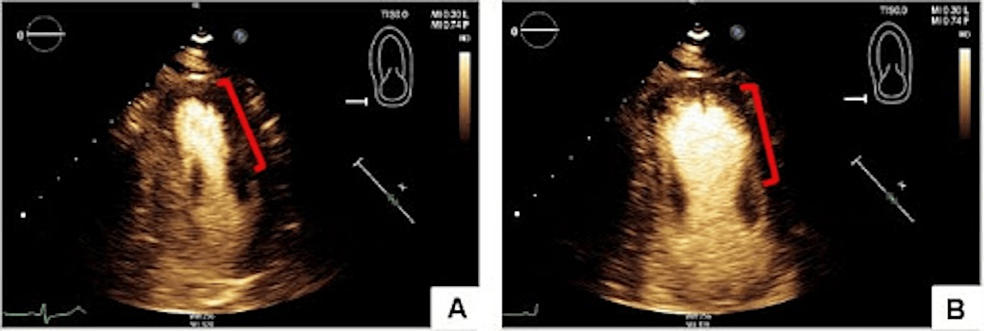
Echocardiography in apical view with perflutren lipid microsphere contrast demonstrating LV chamber in systole (A) and diastole (B). Note the LV ballooning and anterior wall hypokinesis in diastole and systole indicated by the red brackets. LV: left ventricle.
